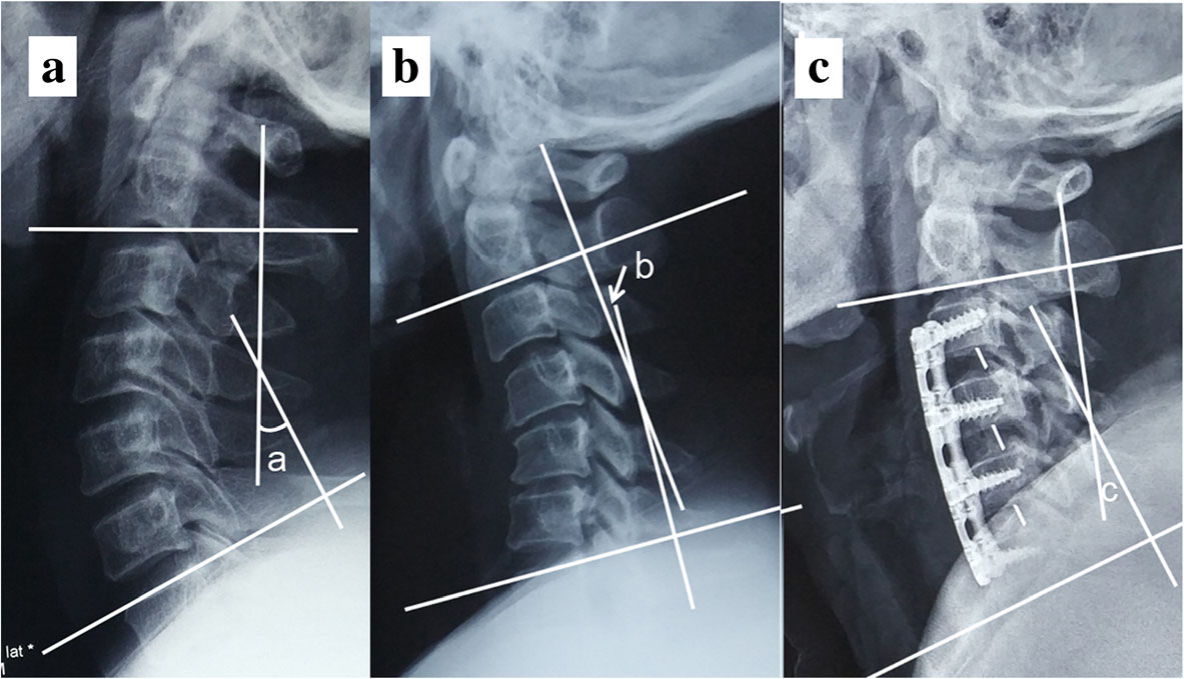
The cervical lordosis (CL), namely as C2–7 Cobb angle, was calculated by measuring the angle between the C2 subvertebral endplate plane and the extension line of C7 subvertebral endplate plane at preoperation (A, B) and postoperation (C) on lateral radiographs. Anterior convex was positive (a) and posterior convex was negative (b)
